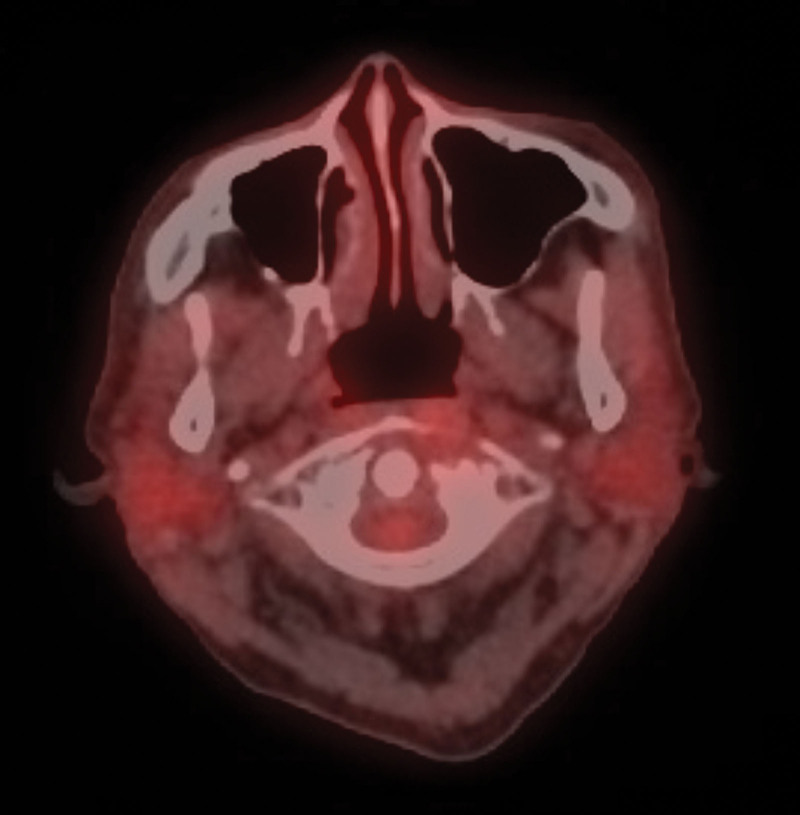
PET CT showed no evidence of FDG-avid lesion. CT = computed tomography, FDG = fluorodeoxyglucose, PET = positron emission tomography.
